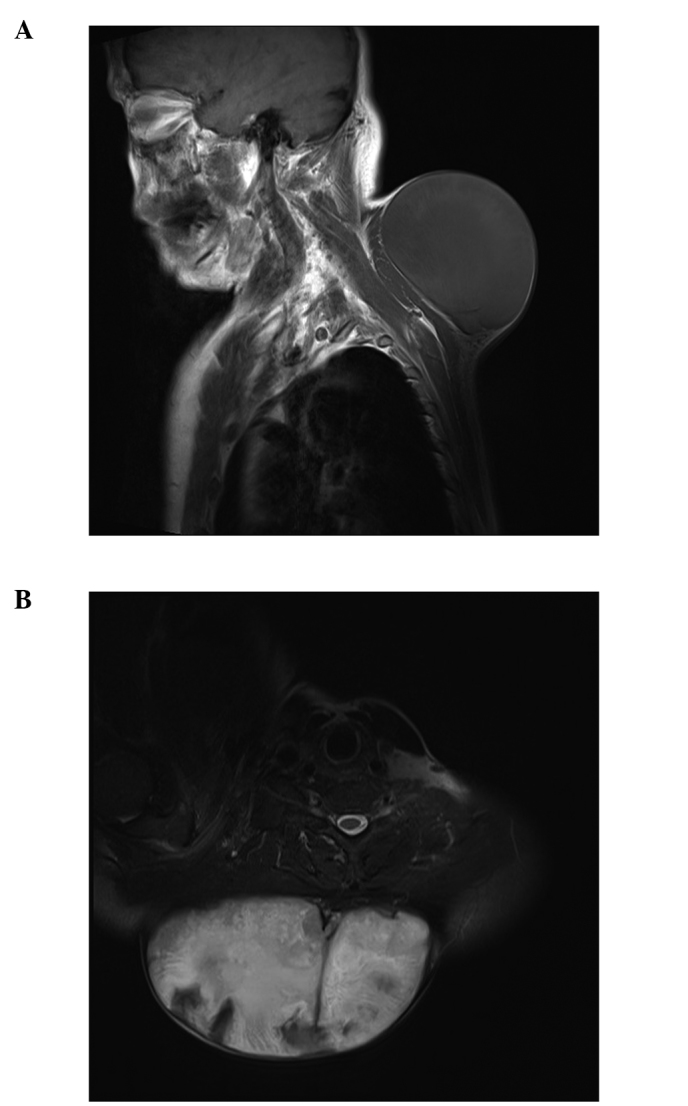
(A) Sagittal T1-weighted MRI revealed a homogenous low intermediate signal intensity mass, surrounded by a low signal intensity well-marginated capsule in the subcutaneous fat of the posterior neck. (B) Axial T2-weighted MRI images showed an oval mass with high signal intensity and debris with low signal intensity at the surrounding capsule.
